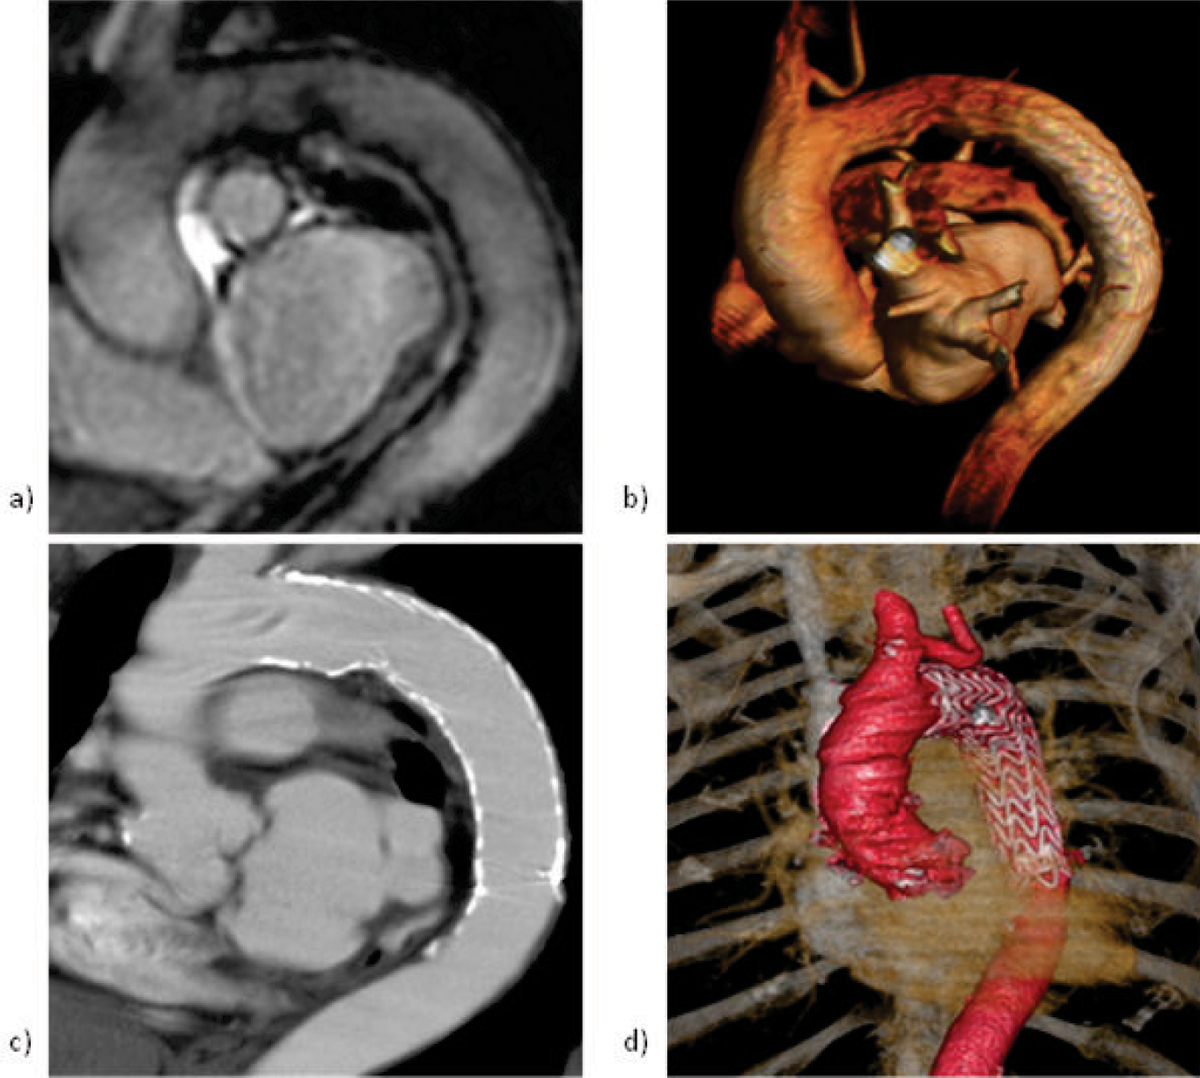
Comparison MRI and CT: a,c) parasagittal two-dimensional and b,d) volume-rendered view of the aortic arch and the thoracic descending aorta.
